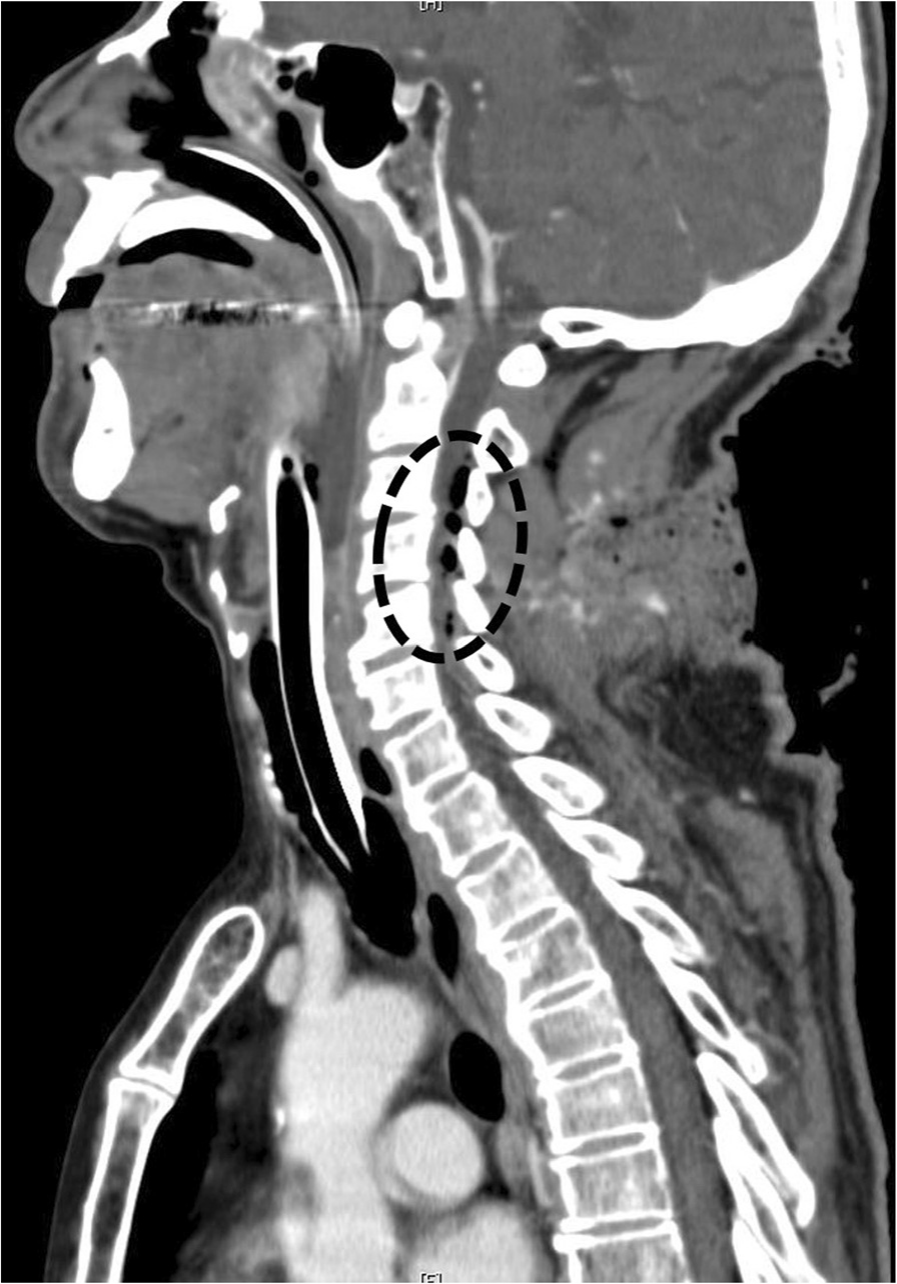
Computed tomography scan showing air in the spinal canal. The *black circle* indicates air in the spinal canal, suggesting dura mater perforation
